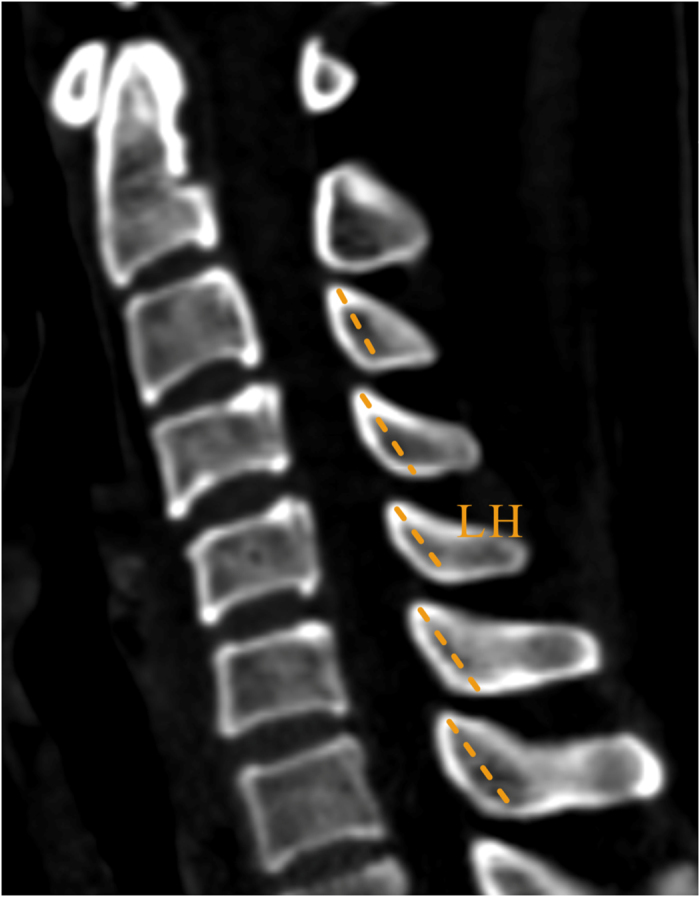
Measurement of the lanimae of lower cervical spine on CT image. In the sagittal plane, laminar height measurement (LH).
